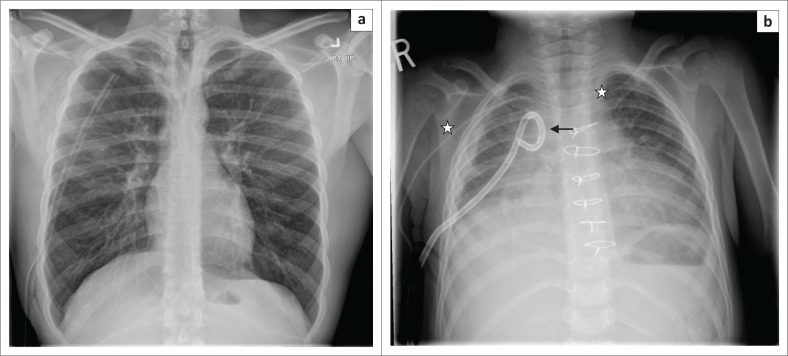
(a) Chest radiograph showing an intercostal drainage (ICD) tube introduced for pneumothorax; (b) a pigtail catheter (arrow) in the right thorax inserted for pleural effusion. Additionally, a malpositioned right peripherally inserted central venous catheter (PICC) [star] is noted with its tip in the left brachiocephalic vein.
